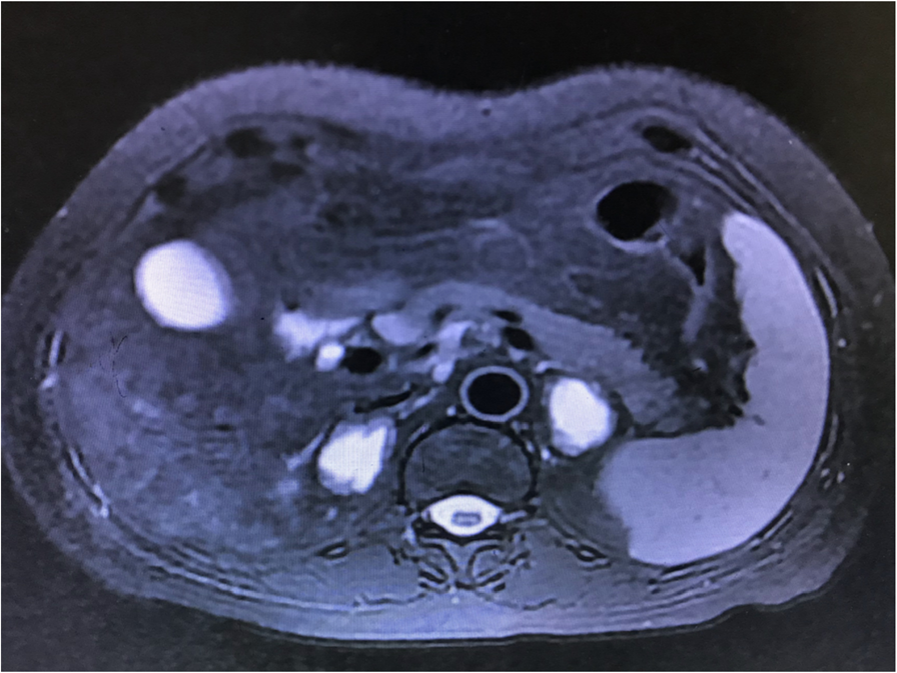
Magnetic resonance imaging (axial section, T2 weighed) on postoperative day 33 displayed water signal intensity of long T2 in central regions of bilateral adrenal mass
